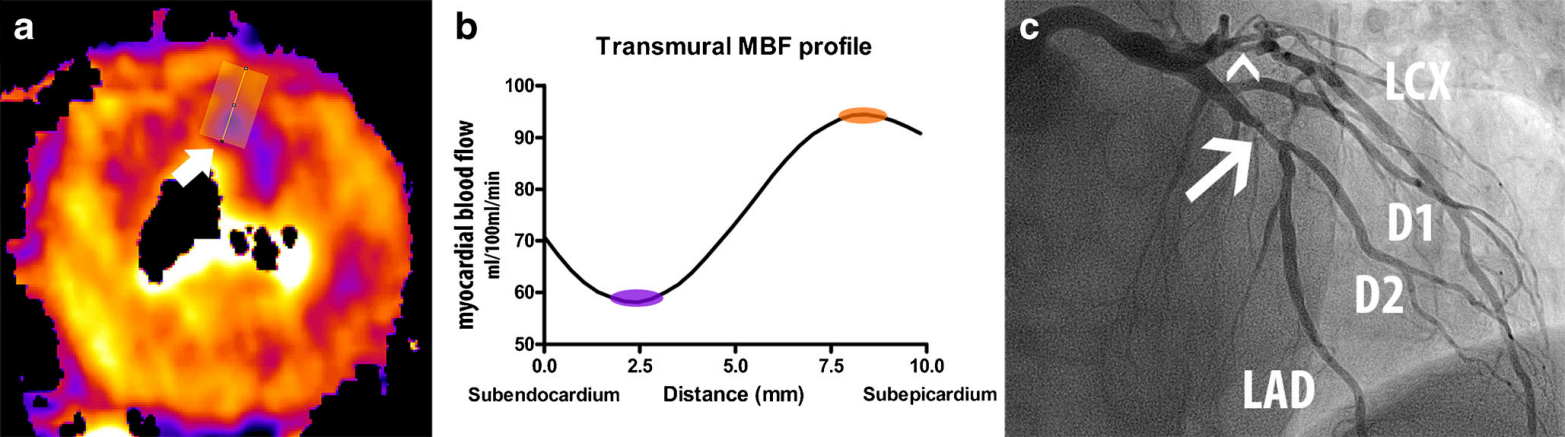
TPR case example: 65-year-old male presenting after exertional collapse. a Short-axis CT-MPI image with the transmural perfusion line placed in the anterior-lateral segment (*white arrow*). b The transmural MBF profile. The subendocardial MBF was 58 ml/100 ml/min (*purple marker*) and 91 ml/100 ml/min in the subepicardium (*orange marker*). The TPR was 0.64 (58/91) and thus considered positive for ischaemia. c Invasive angiography showing a stenosis in the proximal LAD with an FFR of 0.69. A subtotal stenosis was directly stented in the LCX (*arrowhead*); as such no FFR measurement was performed. In panel a however a perfusion defect with a transmural perfusion ratio can be seen in the territory associated with the LCX. The RCA was normal with an FFR of 0.91. *RCA*: right coronary artery, *LAD*: left anterior descending artery, *LCX*: left circumflex artery, *TPR*: transmural perfusion ratio, *MBF*: myocardial blood flow, *FFR*: fractional flow reserve
